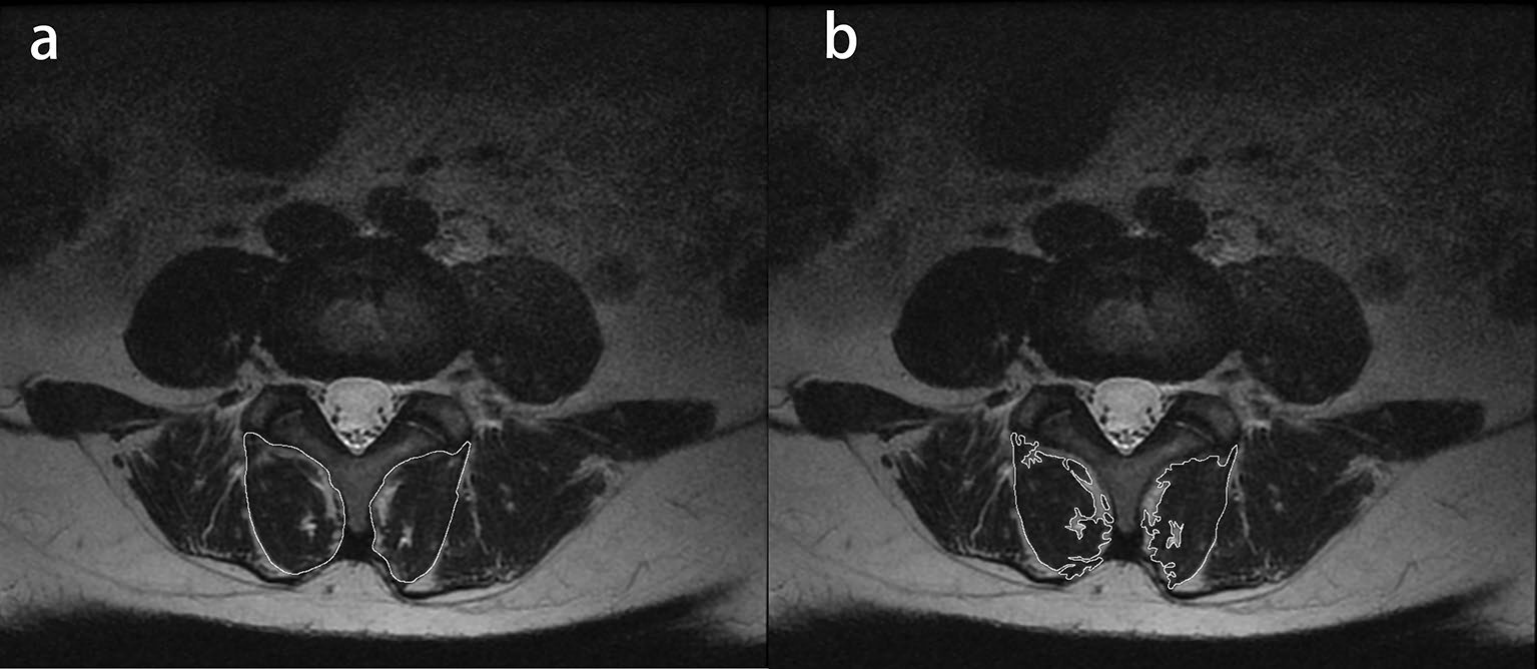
Parameters measured on MRI. (a) Total multifidus muscle cross-sectional area (TCSA). (b) Fat-free multifidus muscle cross-sectional area (FCSA).
